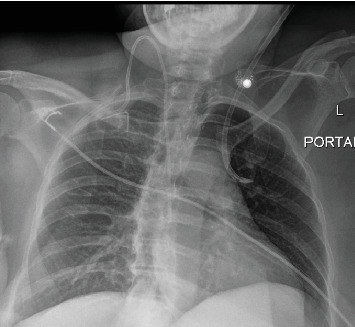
Chest x-ray with concern for catheter malpositioning.
